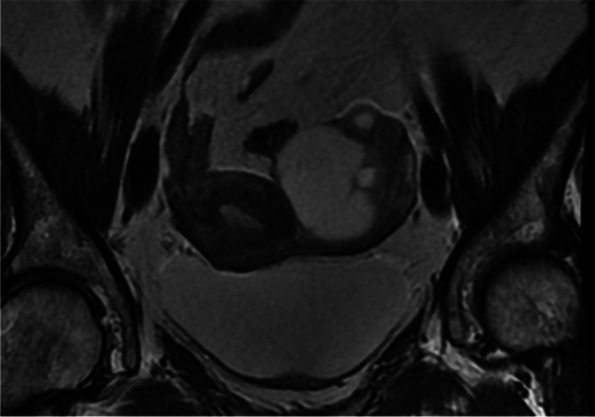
MRI T2-weighted image of solid-cystic lesion
